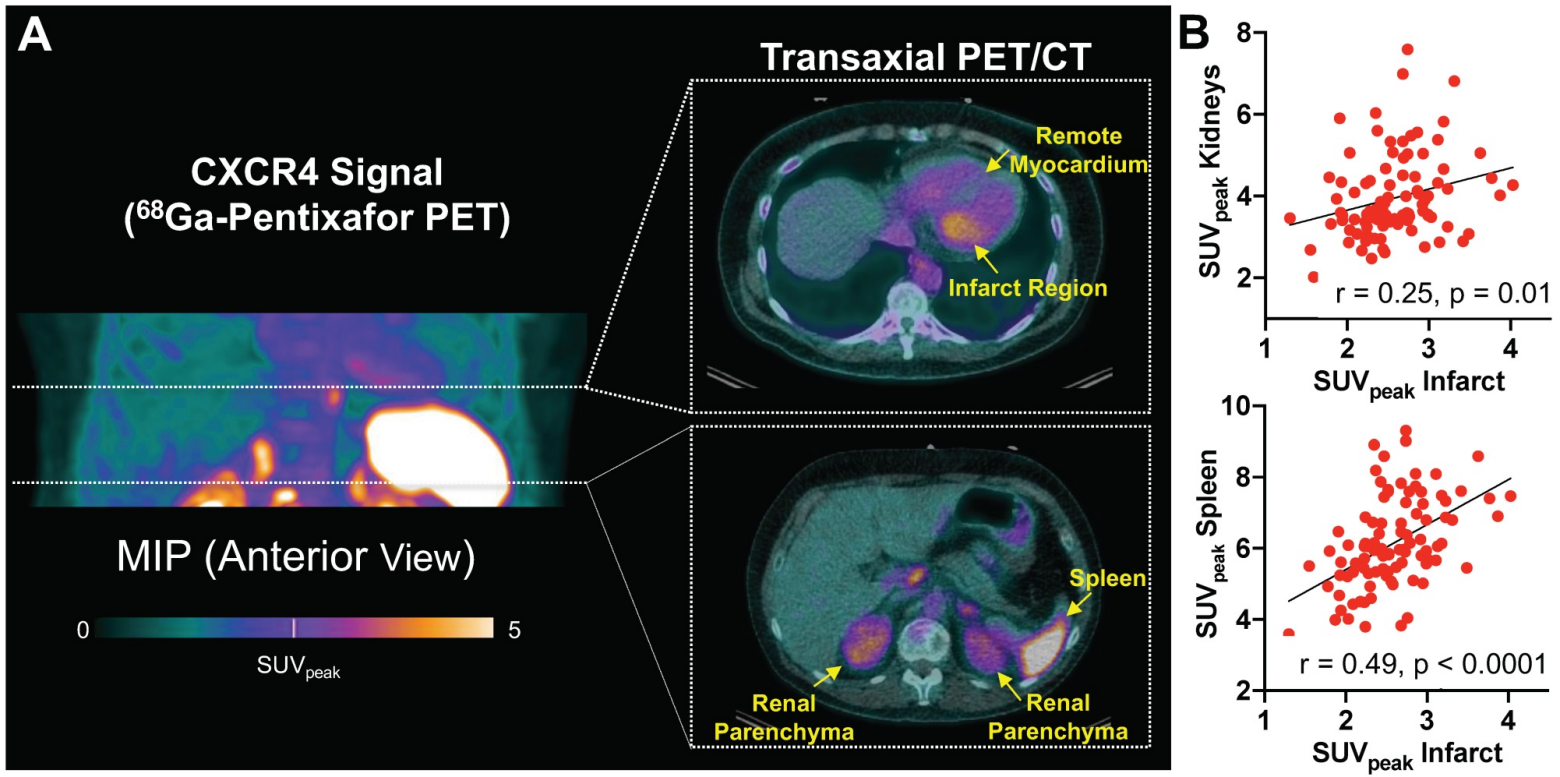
(A) Maximum intensity (MIP) projection of ^68^Ga-Pentixafor PET in a patient early after acute myocardial infarction, along with representative transaxial hybrid PET/CT slices. Spherical volumes of interest are placed in infarct and remote myocardium, spleen, and renal parenchyma. (B) CXCR4 signal derived from infarct territory correlated with uptake in renal parenchyma and spleen. SUV_peak_ = peak standardized uptake value (n = 96).
